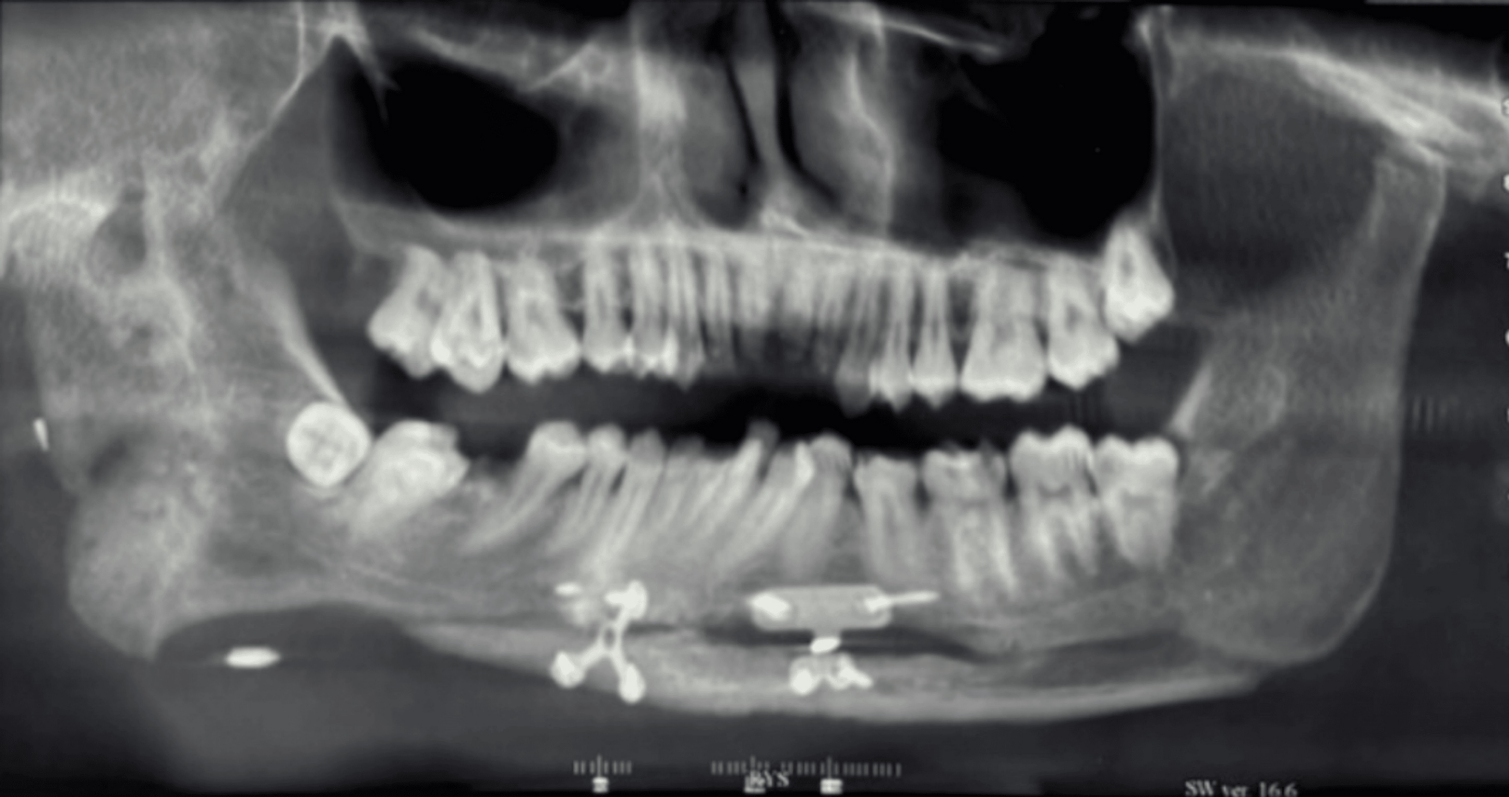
Postoperative cone beam computed tomography radiograph (coronal view) in the third month
